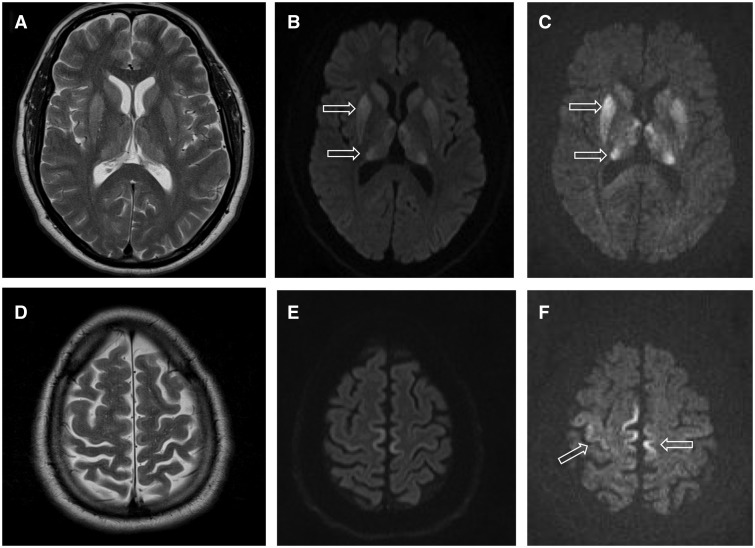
MRI findings in a 46-year-old male patient with iatrogenic CJD due to growth hormone. Basal ganglia and thalamic hyperintensity is seen on T_2_-weighted images (A), DWI at b = 1000s/mm^2^ (B) and (C) DWI at b = 3000s/mm^2^. Cortical hyperintensity in the paracentral lobule bilaterally and right precentral gyrus is not visualized on T_2_-weighted images (D) but is seen on DWI b = 1000s/mm^2^ (E) and (F) is more conspicuous at DWI at b = 3000s/mm^2^ (Patient 12).
